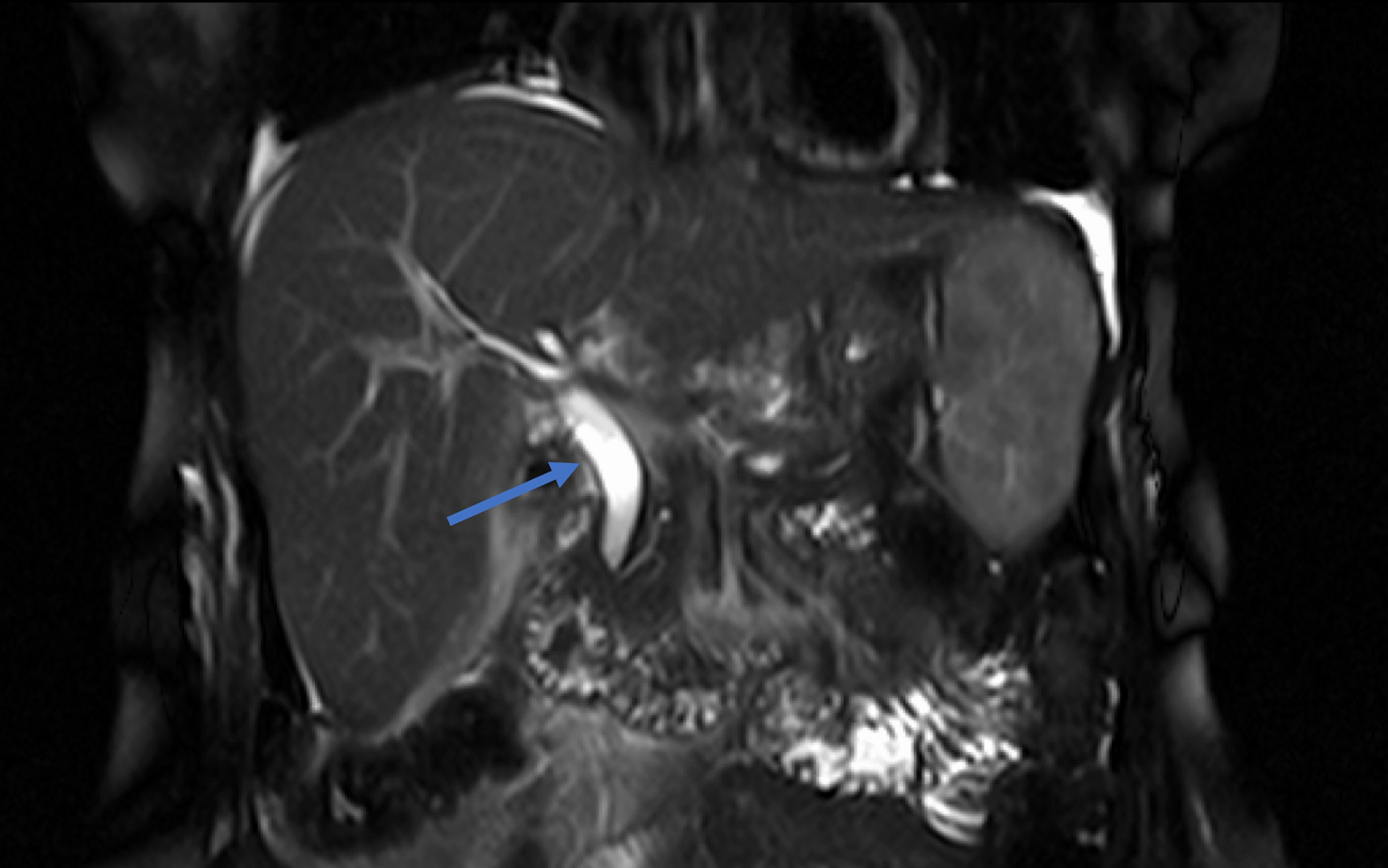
MRI showing dilated common bile duct with no filling defect (blue arrow).
